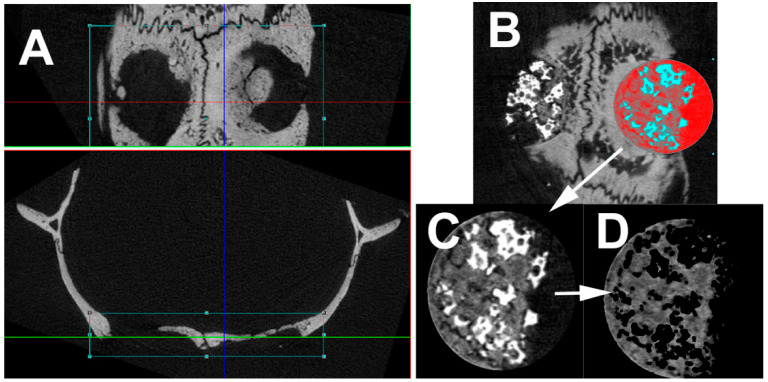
Picture showing the micro-computed tomography (CT) evaluation. (A) First the slices have been placed in correct orientation for reconstruction. (B) Thereafter a circular region of interest (diameter 5 mm) has been placed into the defect. (C) by defining the first and last slice with bone, the volume of interest was defined. (D) Semi-automated evaluation protocol removed very dense regions (Actifuse, NanoBone^®^) as well as air and evaluated the volume of interest with respect to the defined parameters.
